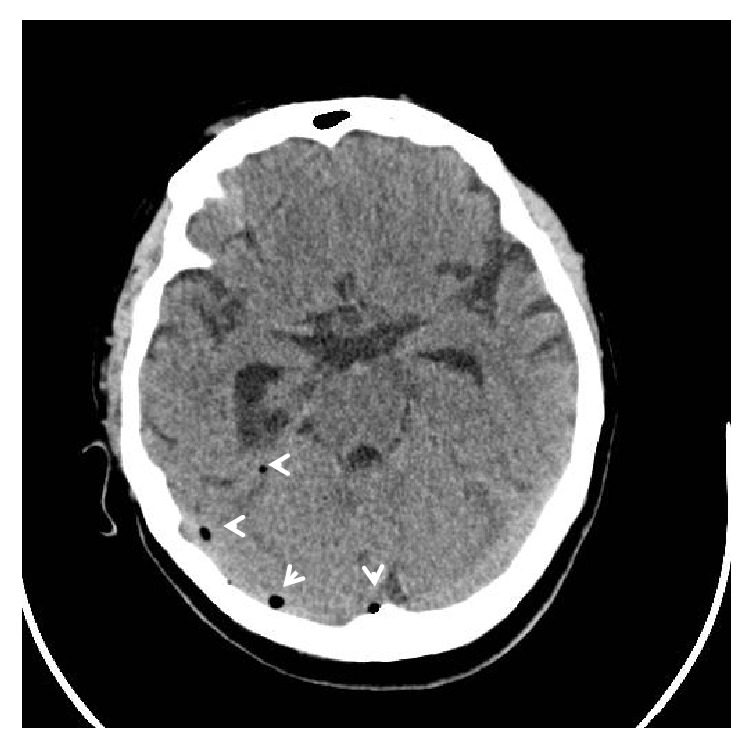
High resolution axial CT showing a focal osseous defect in the posterior mastoid (arrow).
